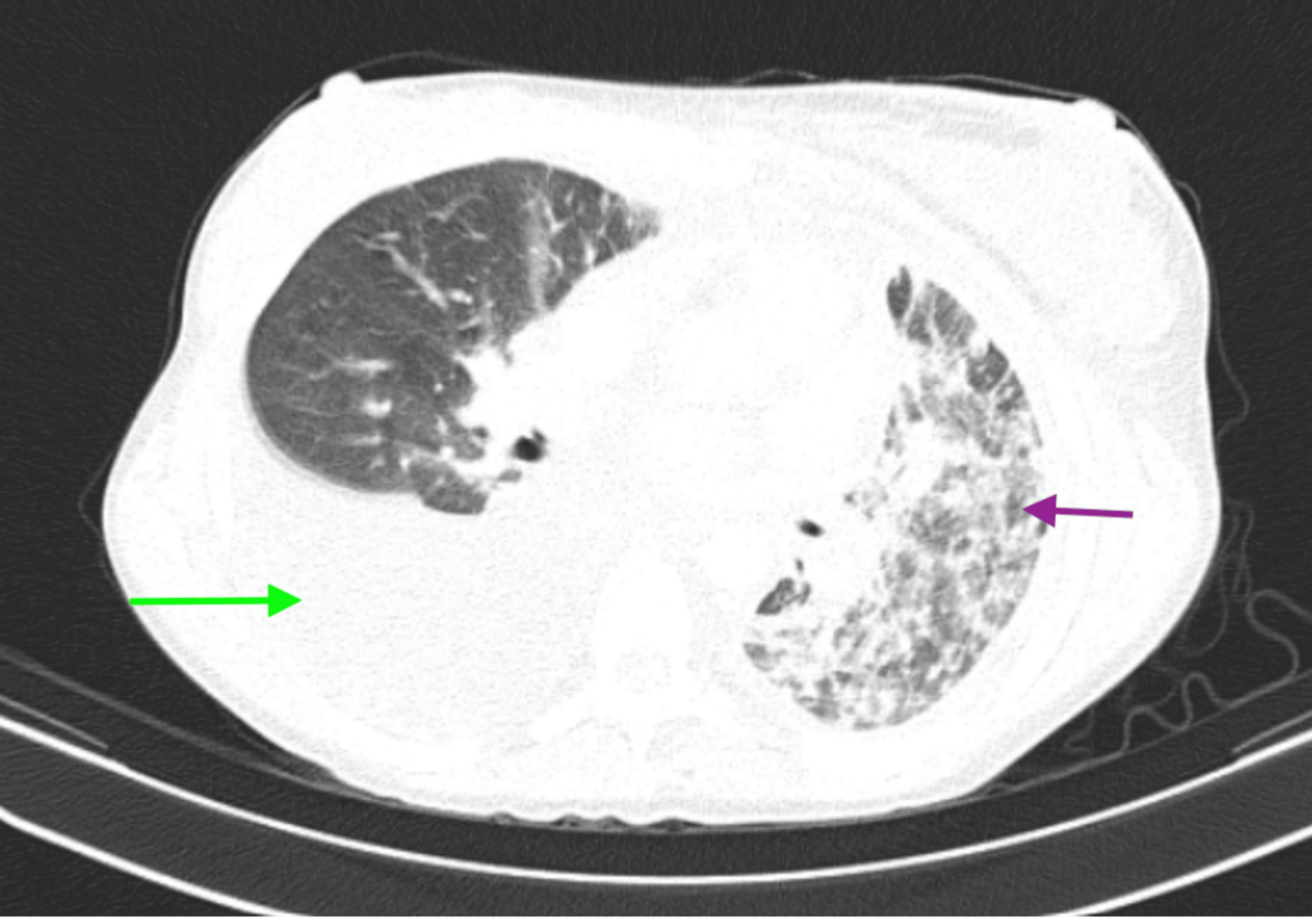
CT scan showing large right-sided pleural effusion (green arrow) and abnormal left-sided parenchyma (purple arrow).
